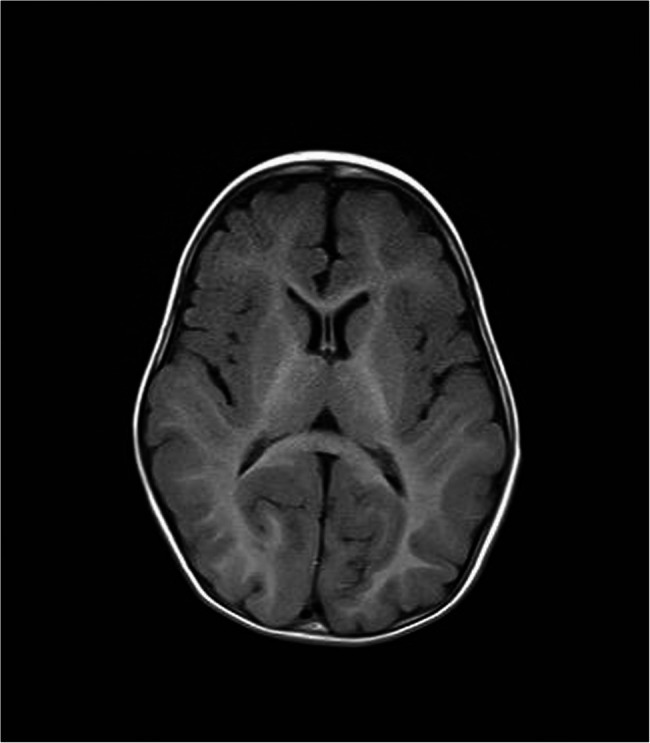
Axial view of brain MRI from patient C-01
